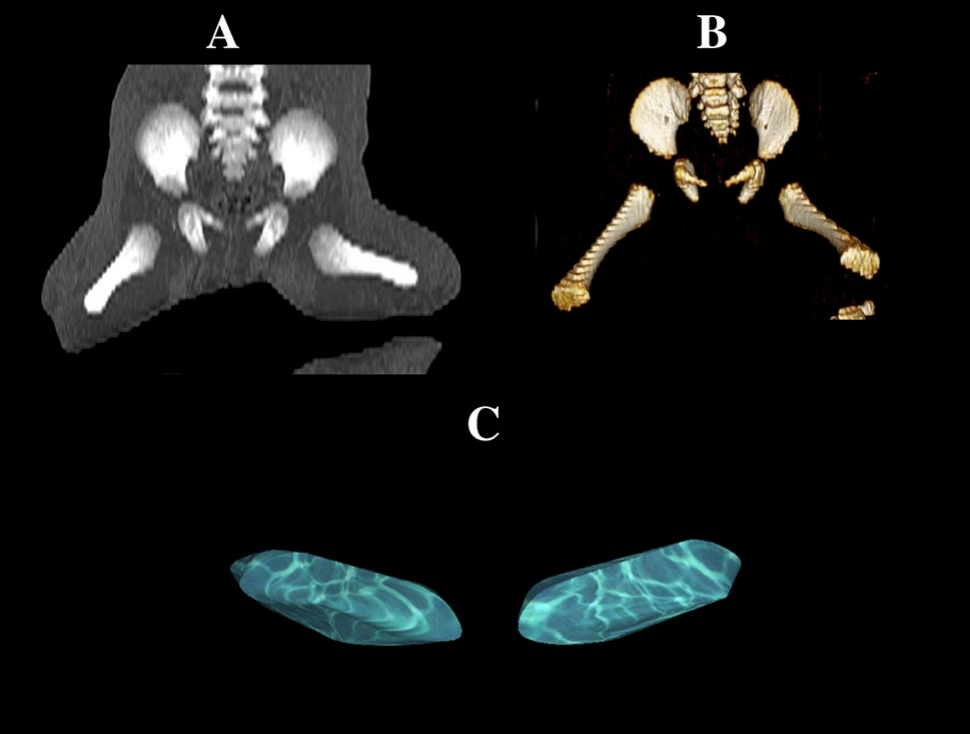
CT of the pelvis of the human fetus in the frontal projection (a), 3D reconstruction of the pelvis in the frontal projection (b), and primary ossification center of the pubis (c) using Osirix 3.9
